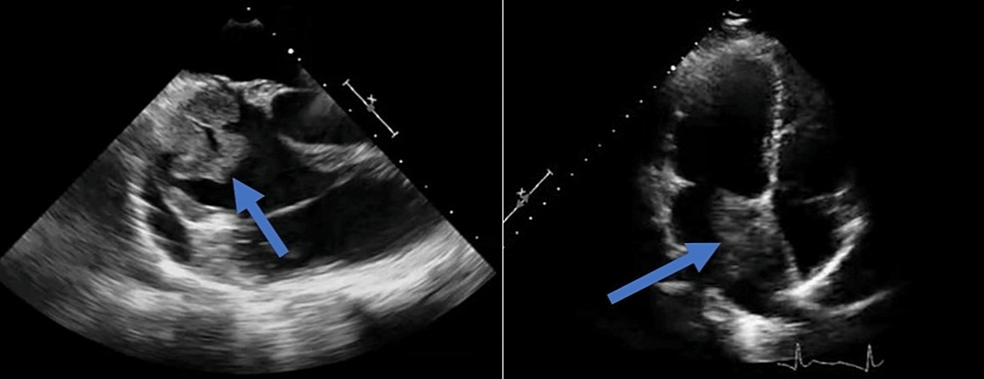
Echocardiogram revealing intracardiac lesion in the right atrium.
